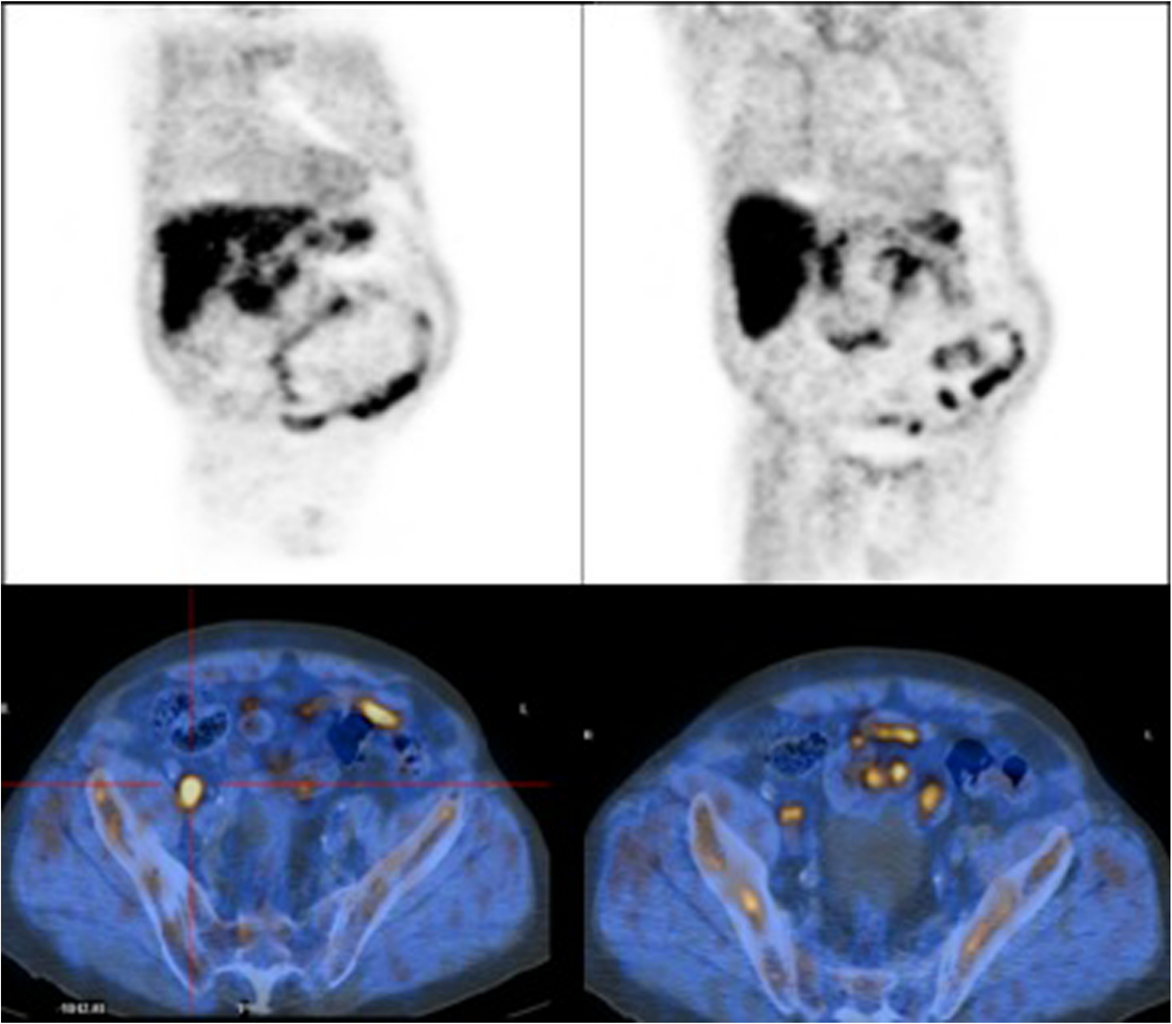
Positron emission tomography–computed tomography of patient 2
